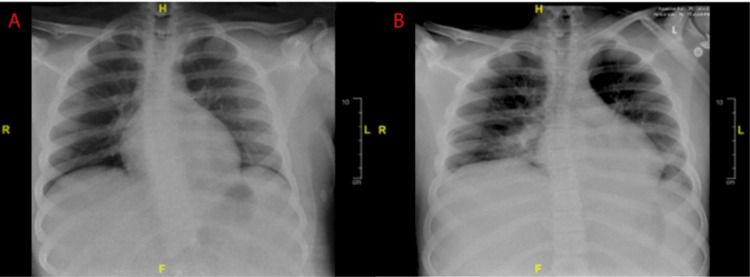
Chest x-ray Chest x-ray taken on day one displays symmetric lung aeration with mildly low lung volumes and crowding of central markings with no airspace disease or pleural disease abnormality identified (A). Chest x-ray taken on day six displays lower lung volumes with slightly increased bibasilar opacities. Left greater than right with partial obscuration of the left hemidiaphragm (B).
